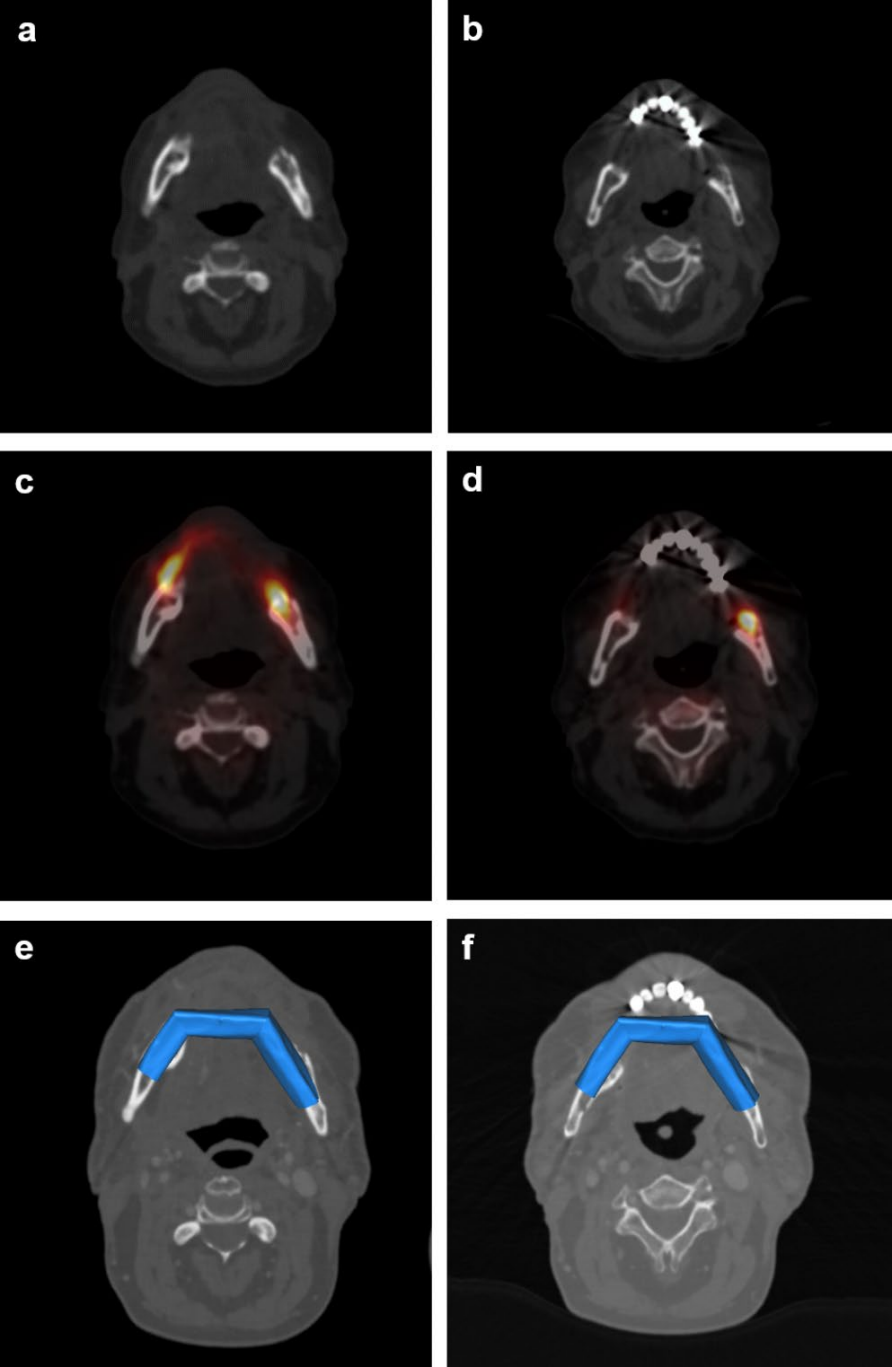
Determination of the right-sided (a, c, e) and left-sided (b, d, f) bony resection margins using low-dose computed tomography (CT) scans (a, b) and single-photon emission computed tomography (SPECT) scans (c, d). The osteotomy planes were defined at 2 mm away from abnormal tracer accumulation in SPECT-CT. Virtual planning of primary mandibular reconstruction (e, f) with a vascularized fibula flap with three segments (blue)
